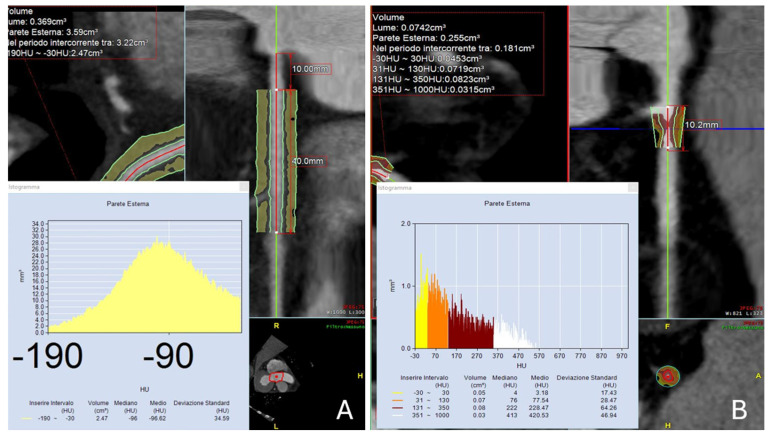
pFAI evaluation on CT analysis. (A) Plaque in the right coronary artery showing normal pFAI. (B) High-risk coronary plaque in the left anterior descending artery showing elevated pFAI.
